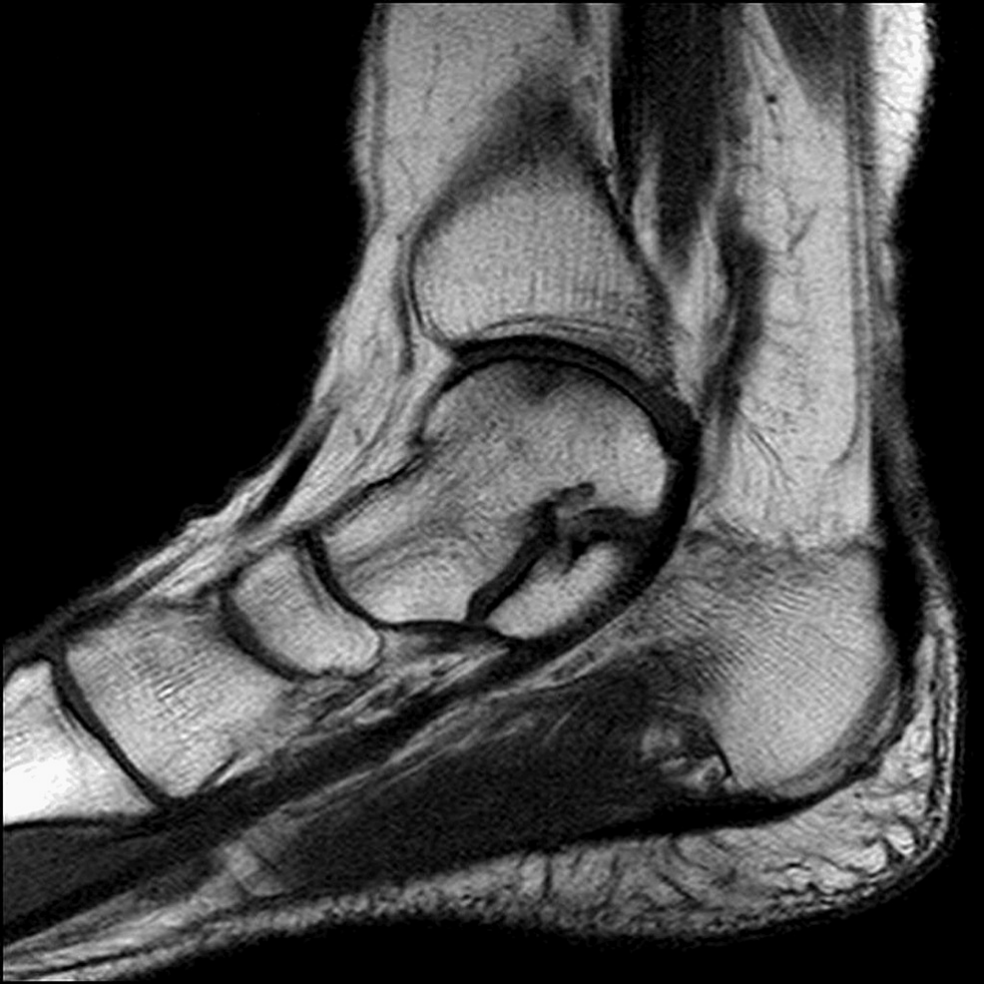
Talus medial osteochondral lesion. Preoperative MRI images: axial section
